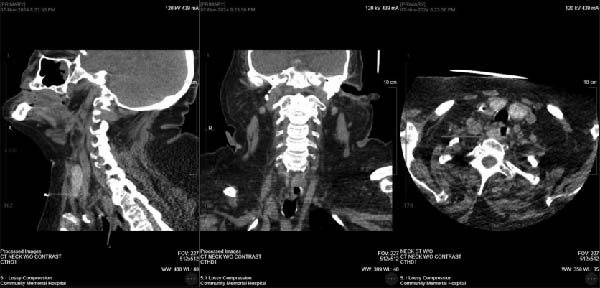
Right paraesophageal mass shown on CT scan.
